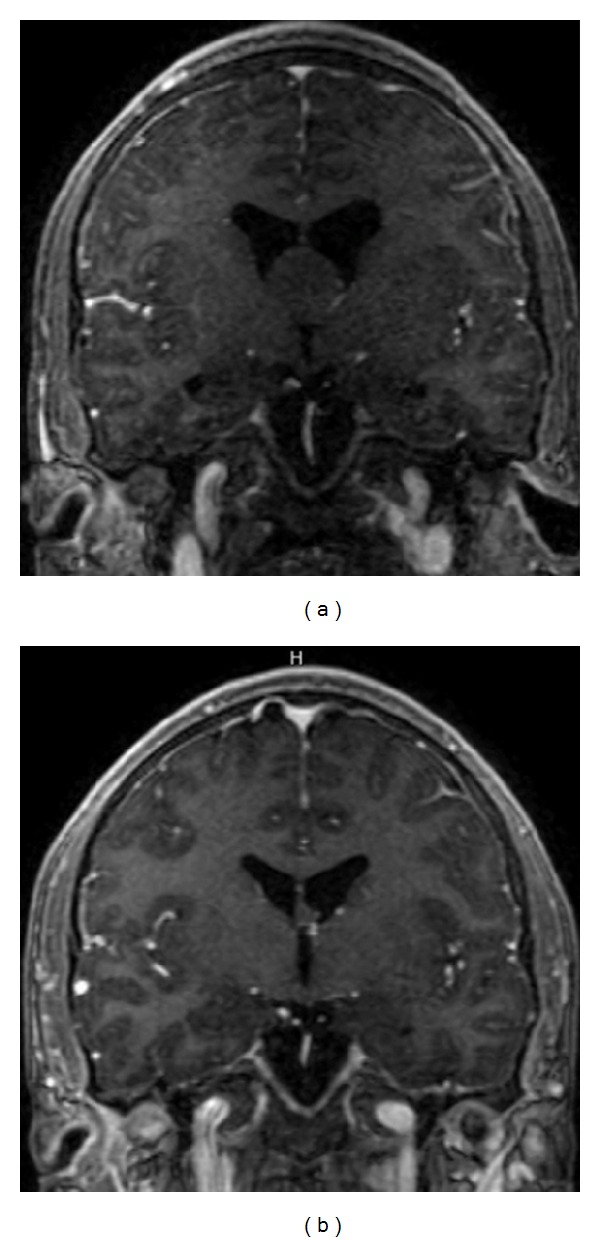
Patient 15, large colloid cyst. (a) Preoperative contrast enhanced coronal T1-weighted magnetic resonance imaging (MRI) showing a lesion with obstructive hydrocephalus. (b) 3-month follow-up MRI shows gross total resection of lesion and resolution of obstructive hydrocephalus.
